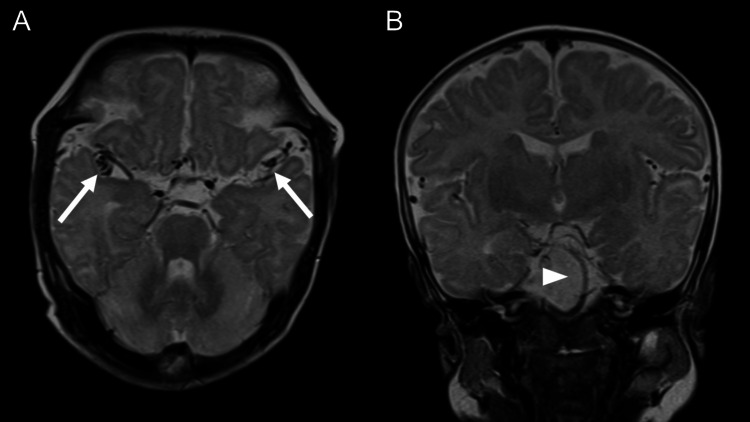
Case 2 (2-month-old male): T2-weighted images (A. Axial level of Sylvian fissure, B. Coronal image). Tortuosity of the middle cerebral artery is depicted in the Sylvian fissure (arrows). The basilar artery is also tortuous in a coronal image (arrowhead).
